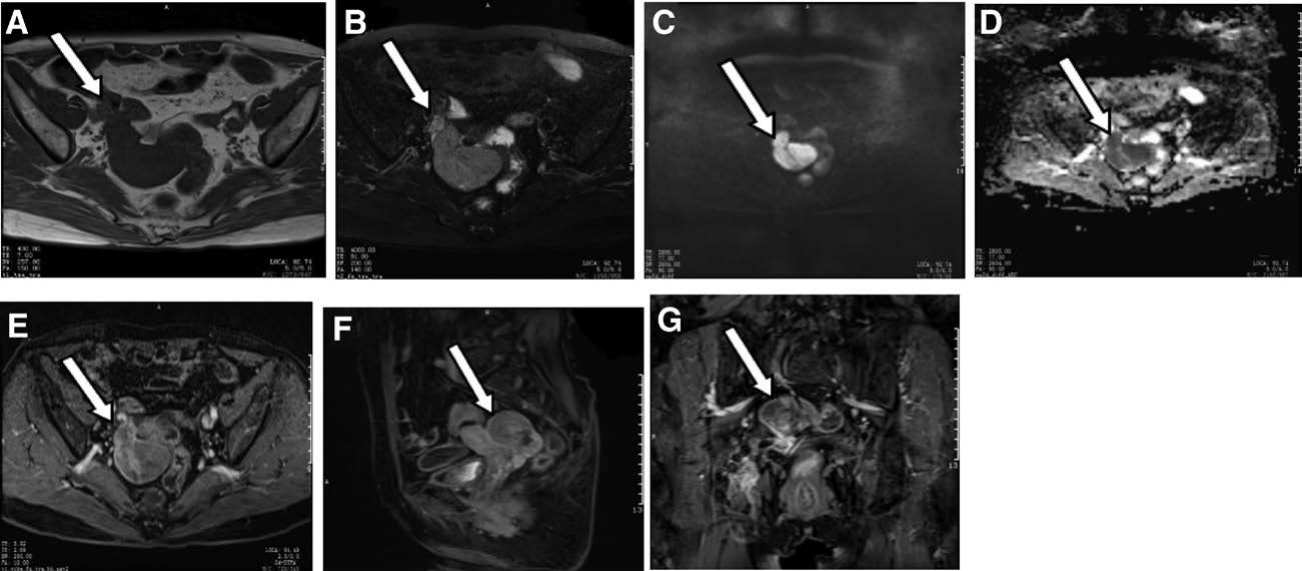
Case 2. Malignant mixed Mullerian tumor of the right fallopian tube. (A) T1WI. (B) FS-T2WI. (C) DWI. (D) ADC. (E–G). Contrast enhancement. ADC = apparent diffusion coefficient, DWI = diffusion-weighted imaging, T1WI = T1-weighted image, T2WI = T2-weighted image.
